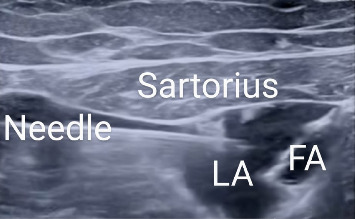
Ultrasound-guided adductor canal block. FA: femoral artery and LA: local anesthetic.
